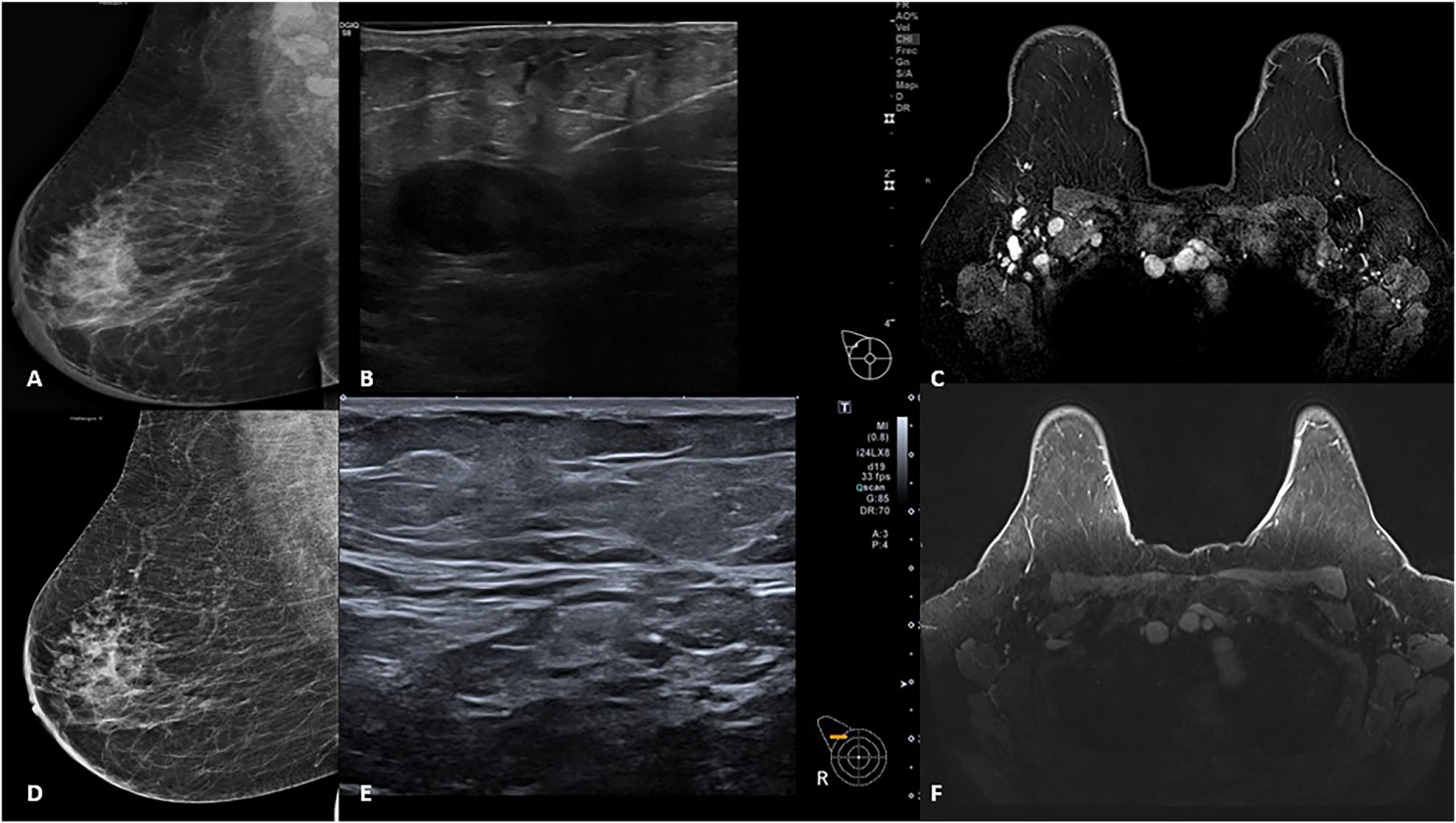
Axillary-rCR Clinical case. HER2+ breast cancer in a 68-year-old woman with invasive ductal carcinoma, grade 3, hormone receptor-negative, HER2+, and Ki-67 of 50%, presenting with more than four suspicious axillary lymph nodes at diagnosis (A–C), who achieved radiological and pCR after NAC (D–F). A Right MLO mammogram showing a retroareolar asymmetric density with enlarged axillary lymph nodes and generalized skin thickening. B Axillary ultrasound demonstrating a pathological lymph node with cortical thickening and fatty hilum replacement (Bedi type 6). C Axial contrast-enhanced T1-weighted fat-saturated MRI showing multiple pathological right axillary lymph nodes. D Follow-up mammogram (MLO view) showing disappearance of axillary lymphadenopathy and resolution of skin thickening. E Axillary ultrasound after NAC revealing lymph nodes with normal morphology, thin cortex, and preserved fatty hilum. F Axial contrast-enhanced T1-weighted fat-saturated MRI showing no residual pathological axillary lymph nodes
